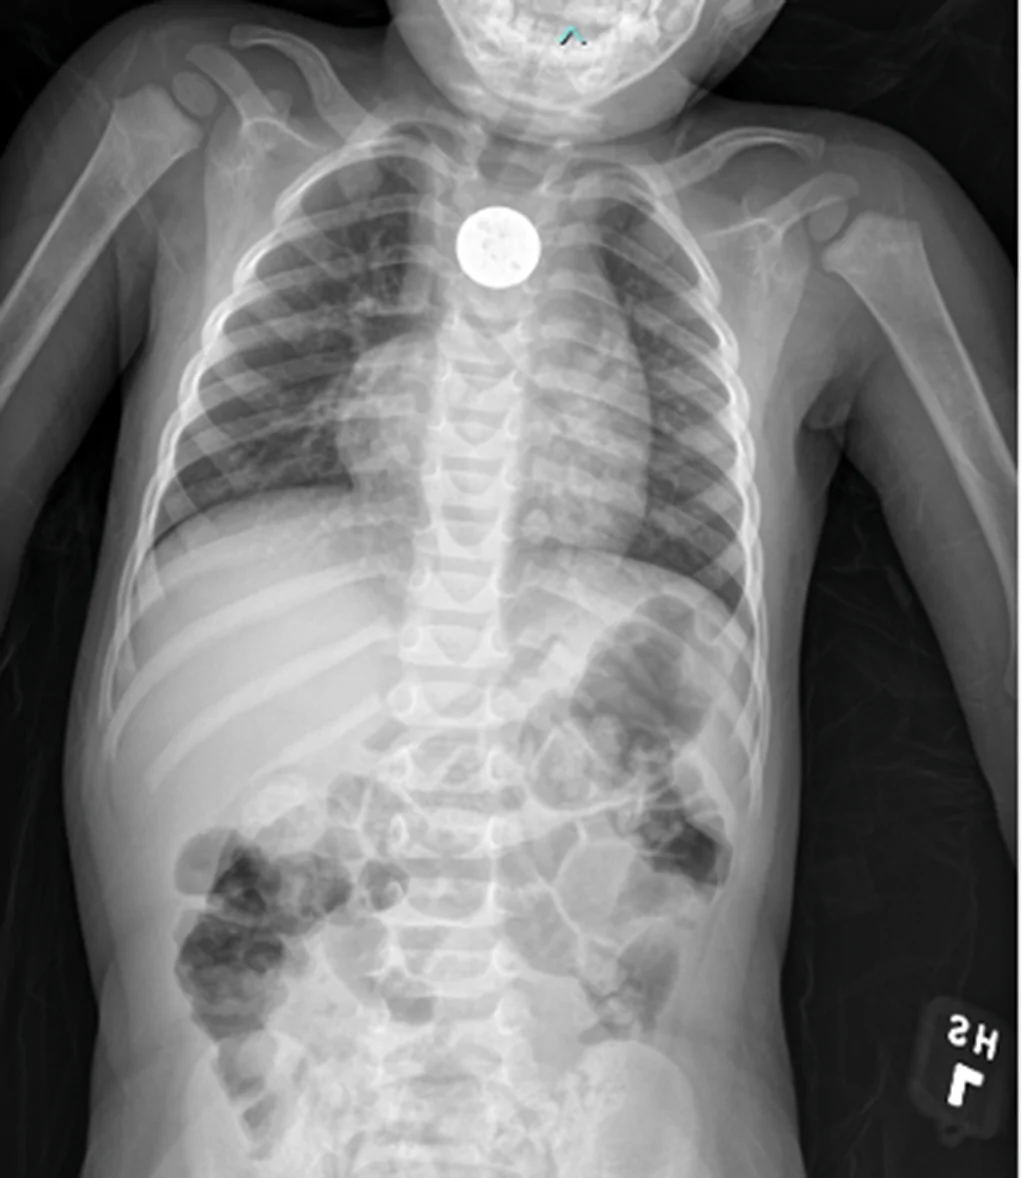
Preoperative radiograph demonstrating an airway foreign body.
